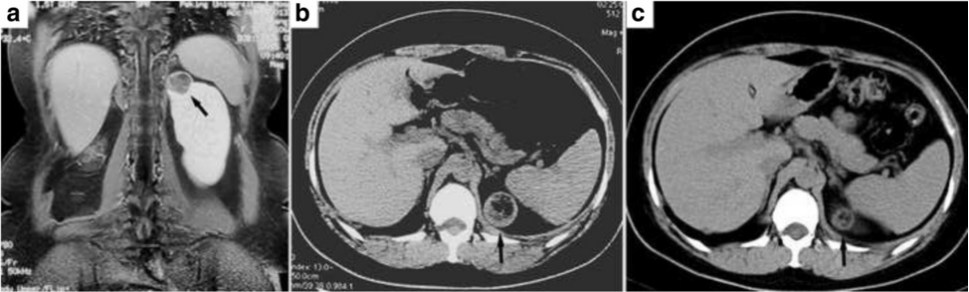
a An abdominal magnetic resonance imaging scan and b an abdominal CT scan showing a round heterogeneous mass of 4 cm in diameter (arrow) on the upper pole of the left kidney. c Eight months after sirolimus therapy, the CT reveals that the mass has shrunk to 2.1 cm in diameter
